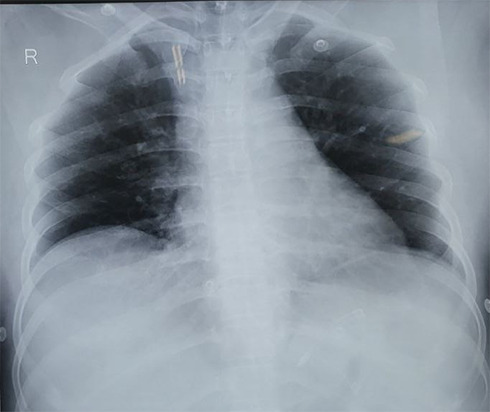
Xray showing bilateral basal opacities
